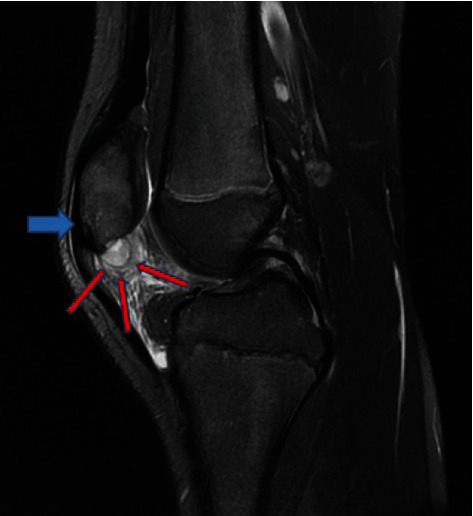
MRI 1. Sagittal T2 fat sat view. Osteomyelitis of the patella (blue arrow) and fat pad infiltration were observed (red arrows).
